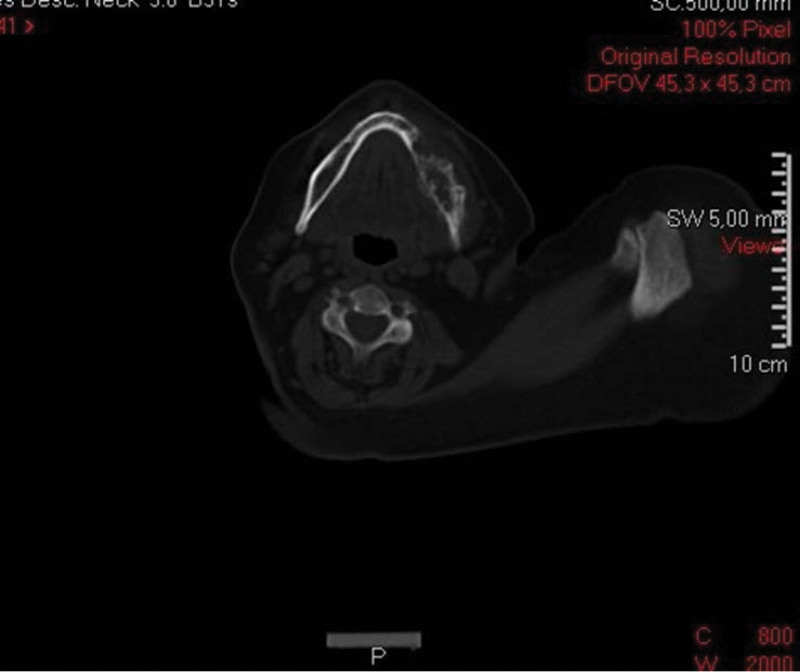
CT scan of the lower jaw. CT = computed tomography.
